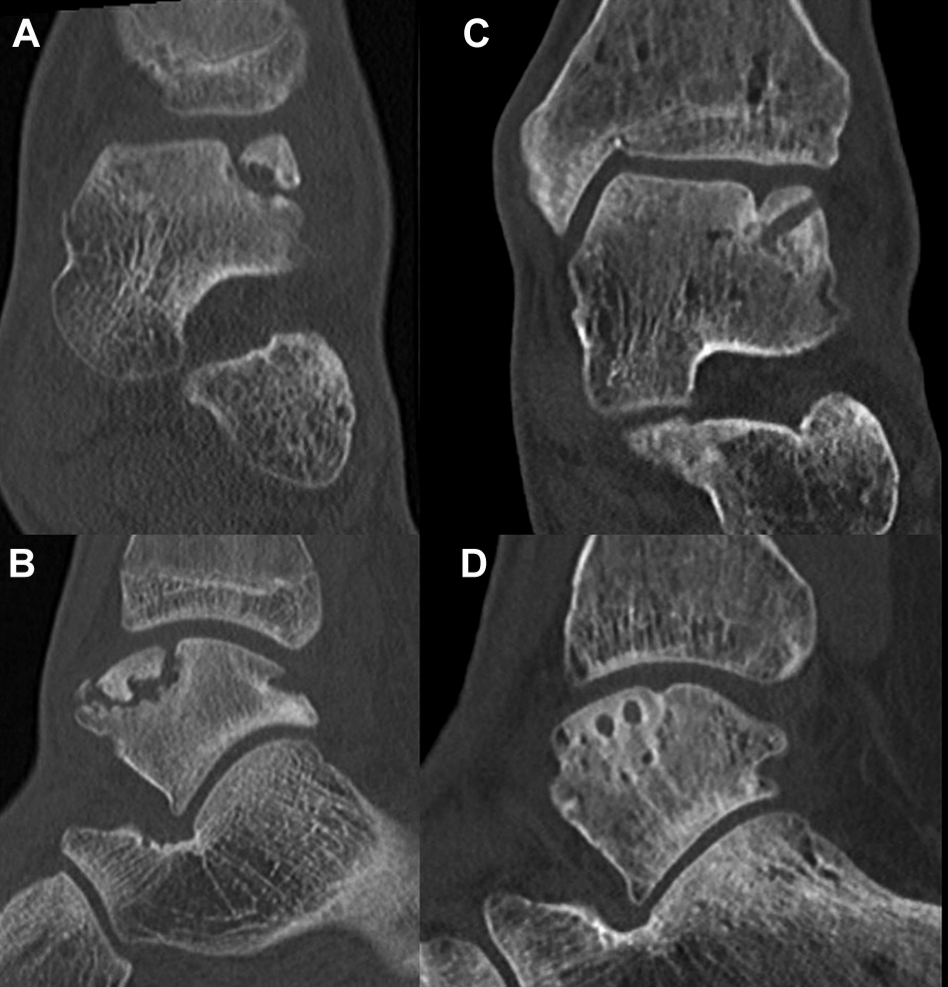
Preoperative coronal (A) and sagittal (B) and 1-year postoperative (C, D) computed tomography images of a 16-year-old male with an anterolateral osteochondral fragment of the talus, who underwent open lift, drill, fill, and fix with 2 bioscrews. The postoperative radiolucencies on the talus are the bioscrews in situ.
